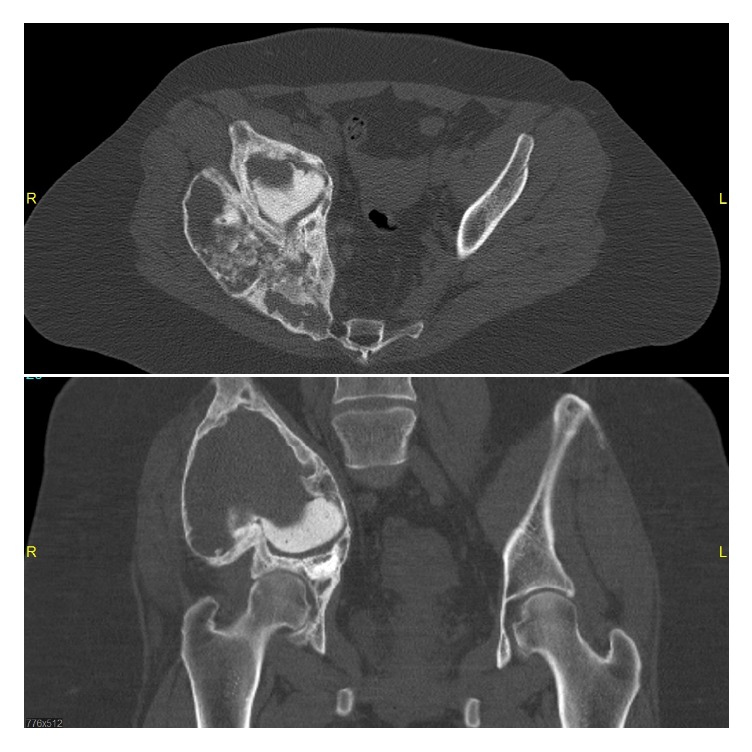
Follow-up CT scan of the pelvis showing stable disease after discontinuation of the second cycle of Denosumab treatment for fifteen months.
